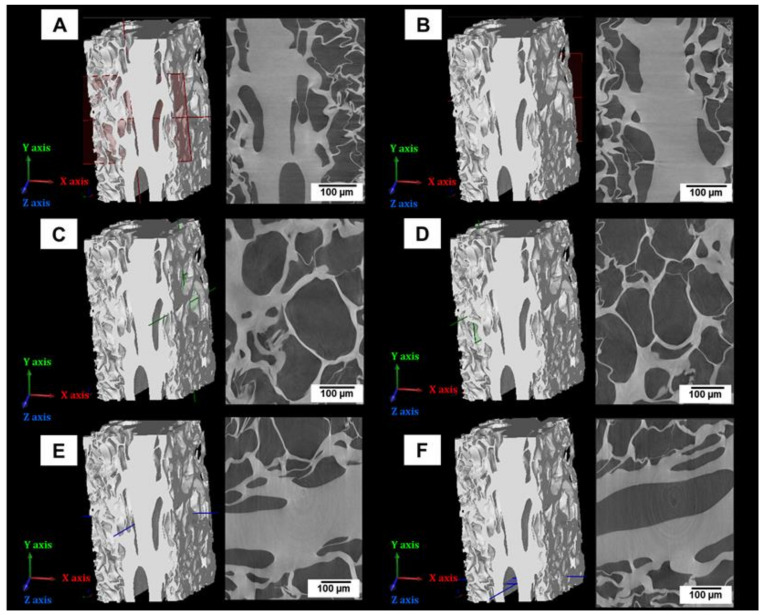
Representative images of orthogonal planes of the lyophilized PVP hydrogel obtained by nano-CT. The images (right side) were acquired in orthogonal planes of the virtual reconstruction (left side): (A,B) XY-plain in red colour, (C,D) YZ plain in green colour and (E,F) XZ-plain in blue colour.
